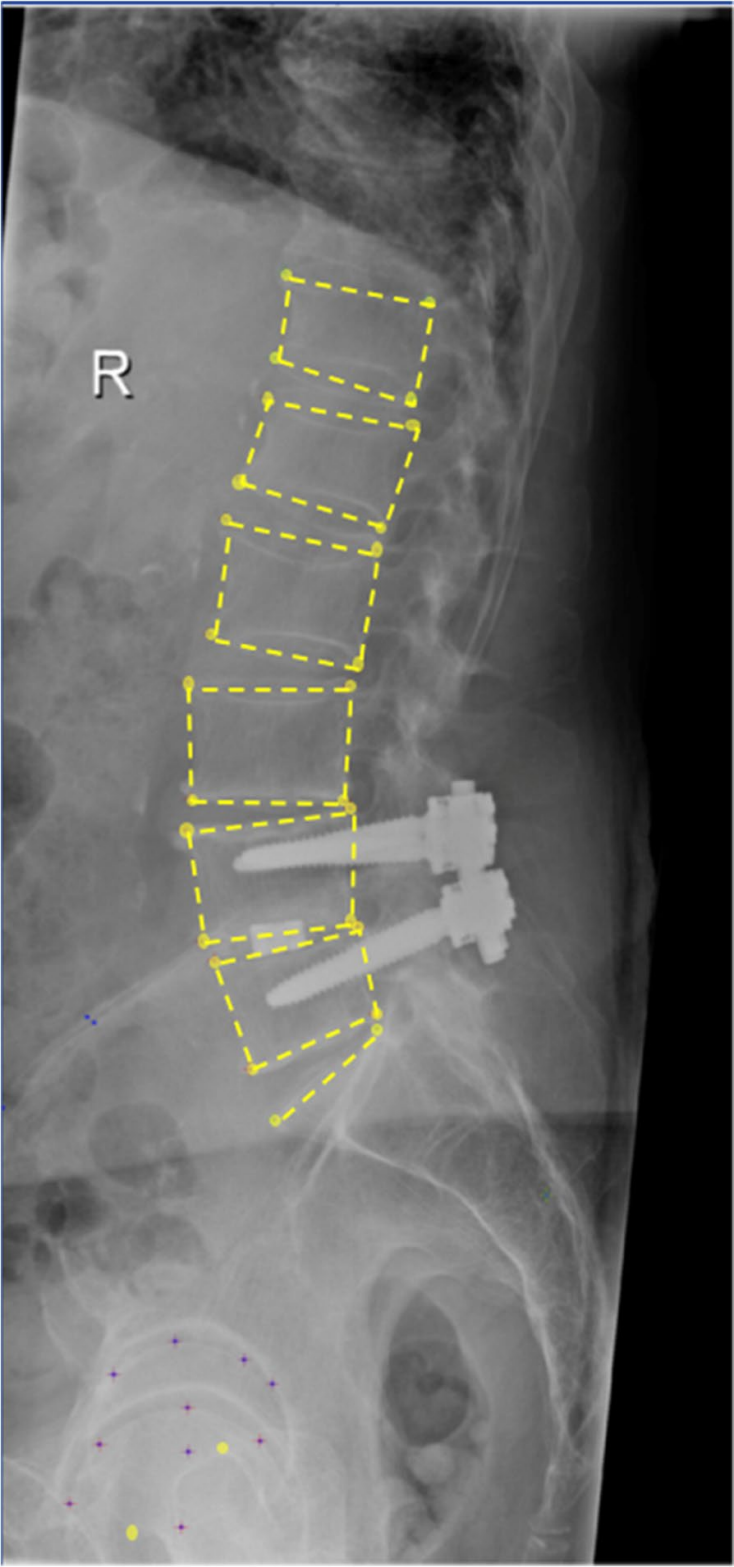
Annotation of the femoral heads, sacral endplate, and vertebral bodies on a lateral x-ray of a patient who had undergone L4/5 spinal fusion
